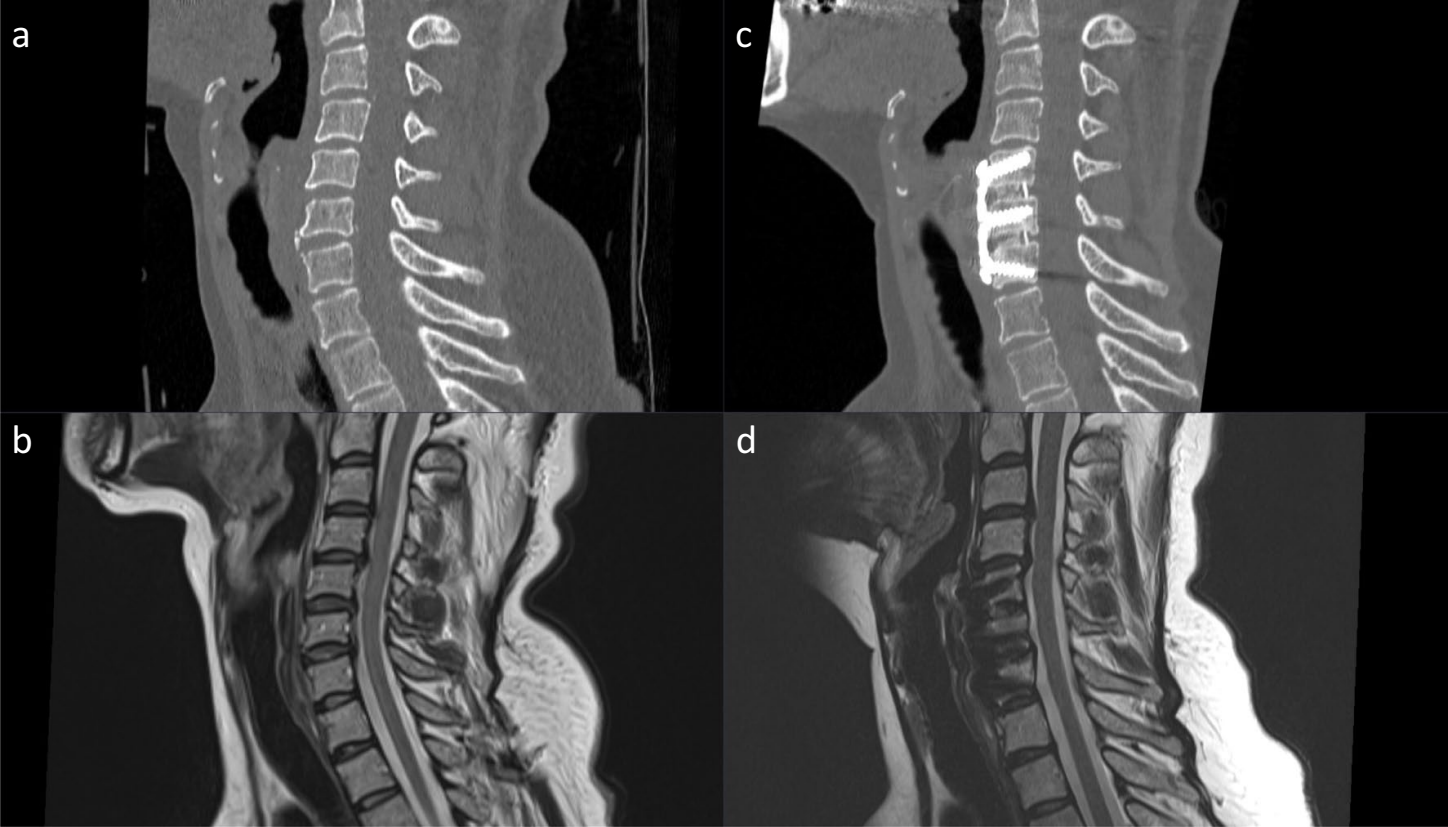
Preoperative CT (A) and MRI (B) as well as postoperative CT (C) and MRI (D) of a patient undergoing a 2-level anterior discectomy and fusion (ACDF)
